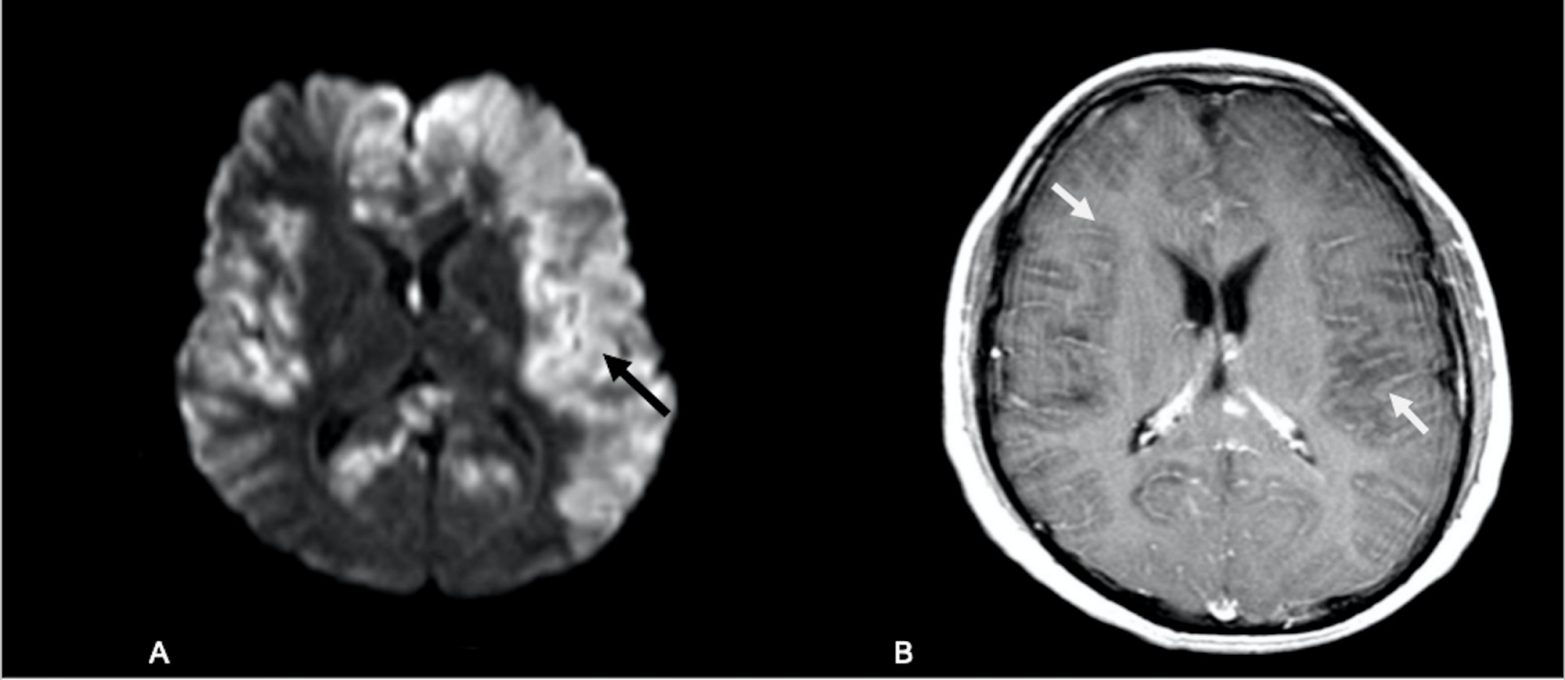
Follow-up brain MRI (A) MRI brain diffusion images demonstrate a marked increase in extent of left greater than right hemispheric diffusion restriction (black arrows). (B) Axial T1 postcontrast images demonstrate new widespread sulcal enhancement (white arrows).
